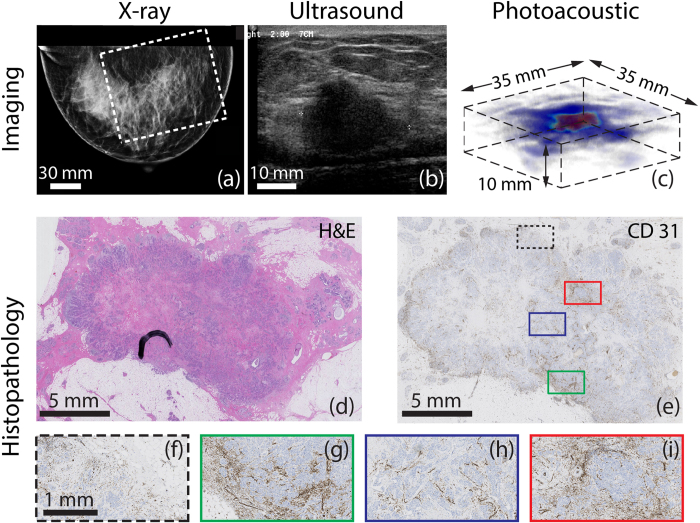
Example of photoacoustic mass-like appearance seen in 44 year old patient (P38, Case 1) with infiltrating ductal carcinoma (IDC). (a) The cranio-caudal x-ray mammogram of the right breast does not show any abnormalities and is judged as BI-RADS 1. The white box indicates the field-of-view (FOV) of the PA image. (b) Ultrasound image of palpable mass, reveals 22 mm highly suspect mass. (For MR image see Fig. 1(a)) (c) A volume rendering of part of the PA data reveals confined high intensity area, which we define as mass appearance. The corresponding CC AIP is seen in Fig. 1(b)
